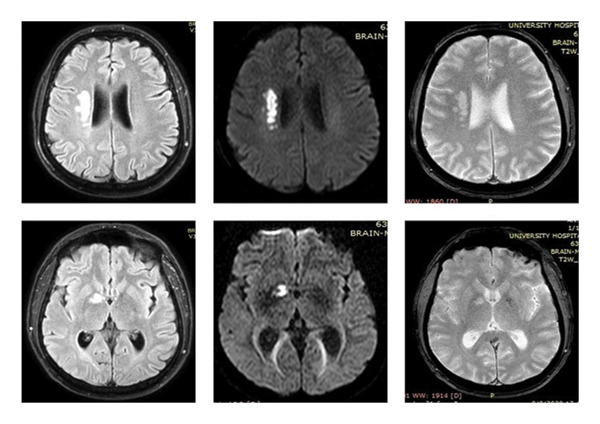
Brain MRI of Patient 1 in FLAIR, DWI, and T2 axial sections, respectively. The upper row shows a subacute ischemic stroke close to the right lateral ventricle with increased signal in all the above sequences. The lower row depicts a similar lesion of the right lenticular nucleus.
